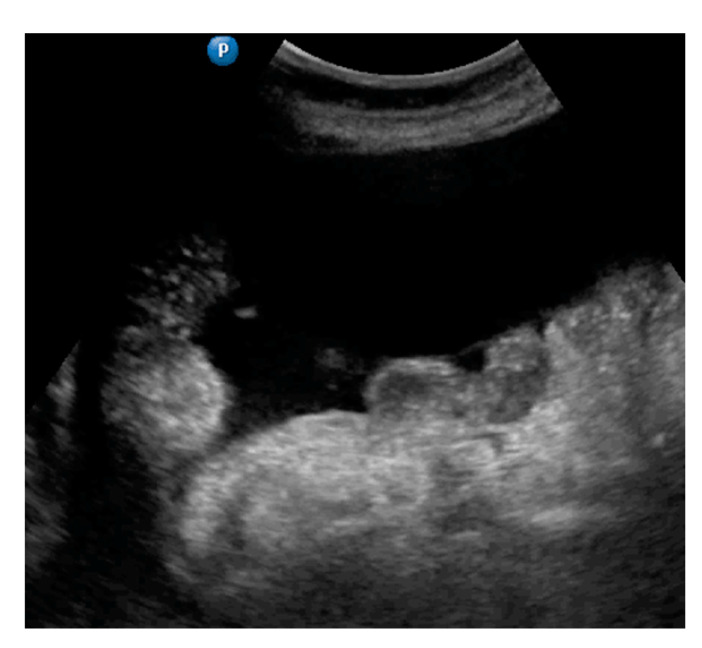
Ultrasound image of ascites.
